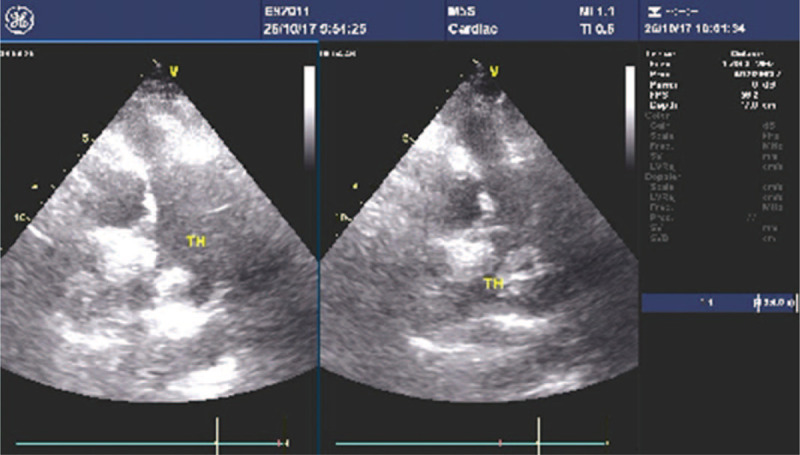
Echocardiography showing a large thrombus in the left atrial appendage after 6 months of anticoagulation with dabigatran.
